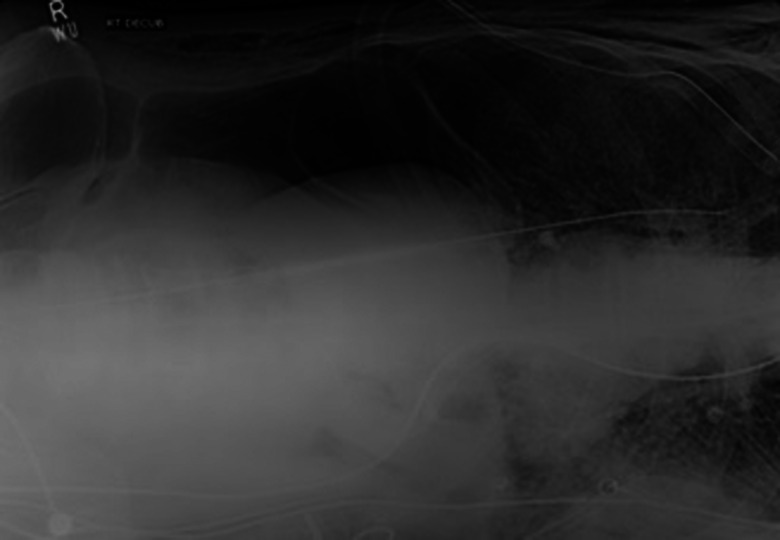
Abdominal x-ray showing extensive pneumoperitoneum.
